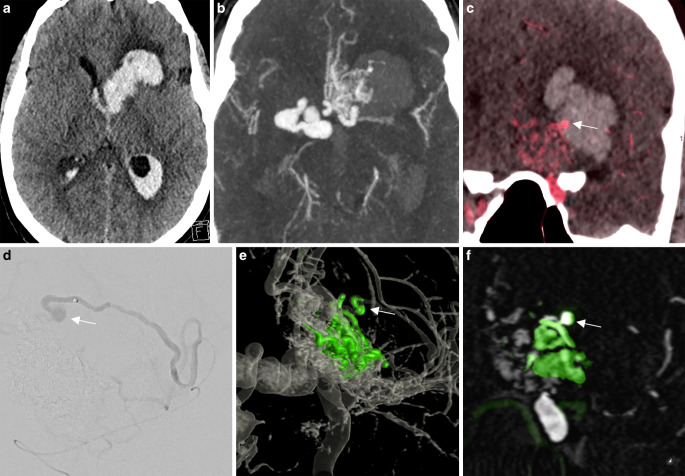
Ruptured arteriovenous malformation (AVM) with an intranidal aneurysm. a Nonenhanced computed tomography (CT) in the axial plane demonstrating a deep left basal ganglia hemorrhage with intraventricular rupture. b Computed tomography angiography (CTA) demonstrating a nidus in the left orbitofrontal cortex with several enlarged venous pouches on the contralateral side overlying the carotid terminus. c Three-dimensional rotational angiography (3DRA) + CT fusion in the coronal plane demonstrating a small intranidal aneurysm (*arrow*) centered in the basal ganglia hemorrhage as the bleeding source. d Selective catheterization of the deep perforating branch supplying the aneurysm (*arrow*). e,f Baseline 3DRA + postembolization 3DRA fusion demonstrating the onyx cast (*green*). Note that the inferior and lateral part of the AVM with the aneurysm (*arrow*) have been embolized. Supplementary video demonstrates the fusion of baseline 3DRA + postembolization 3DRA in axial slides
